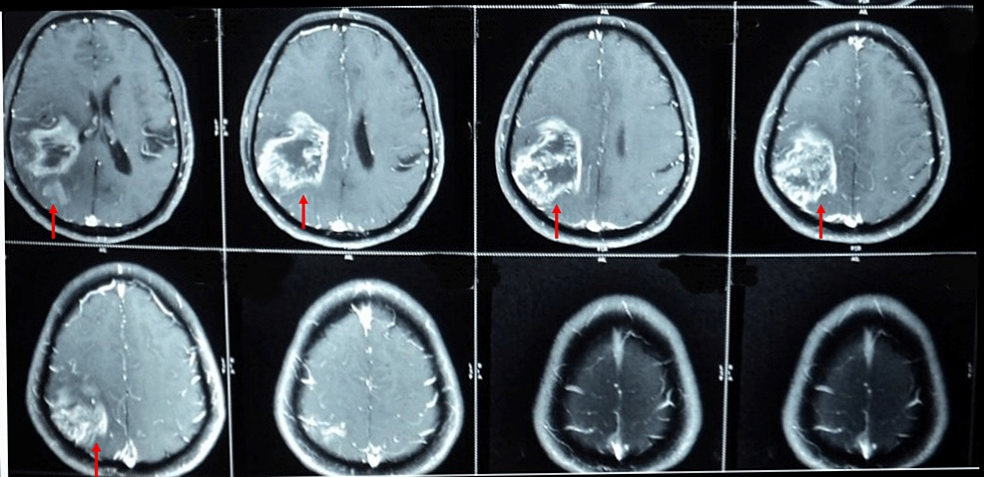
T1W MRI in Case 1 showing relatively well-defined lesion in right parieto-occipital region with peripheral post-contrast enhancement (red arrow). Minimal mass effect to adjacent lateral ventricles and effacement of sulcal spaces; however, no significant midline shift. T1W: T1 weighted
